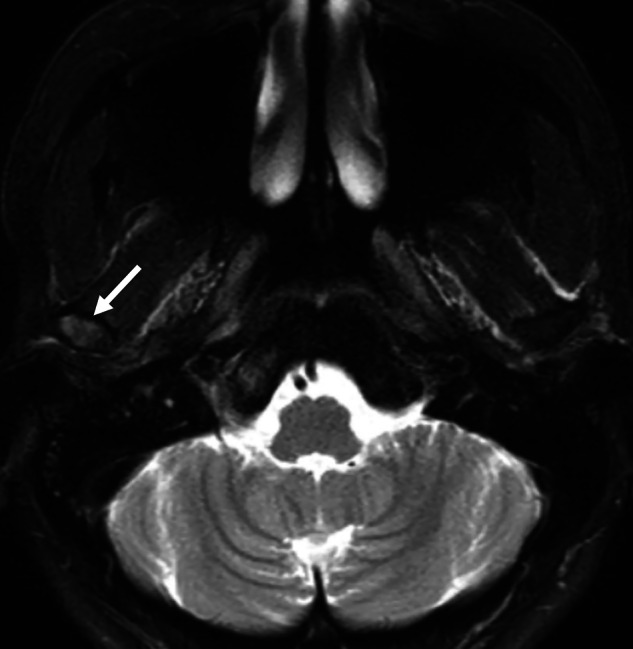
A post-treatment axial fat-suppressed T2W MRI image of a patient with right parotid adenoid cystic carcinoma treated with parotidectomy followed by adjuvant RT 2 years ago. There is an irregular high signal on the right mandibular condyle (white arrow), suggesting marrow inflammation or edema secondary to RT
